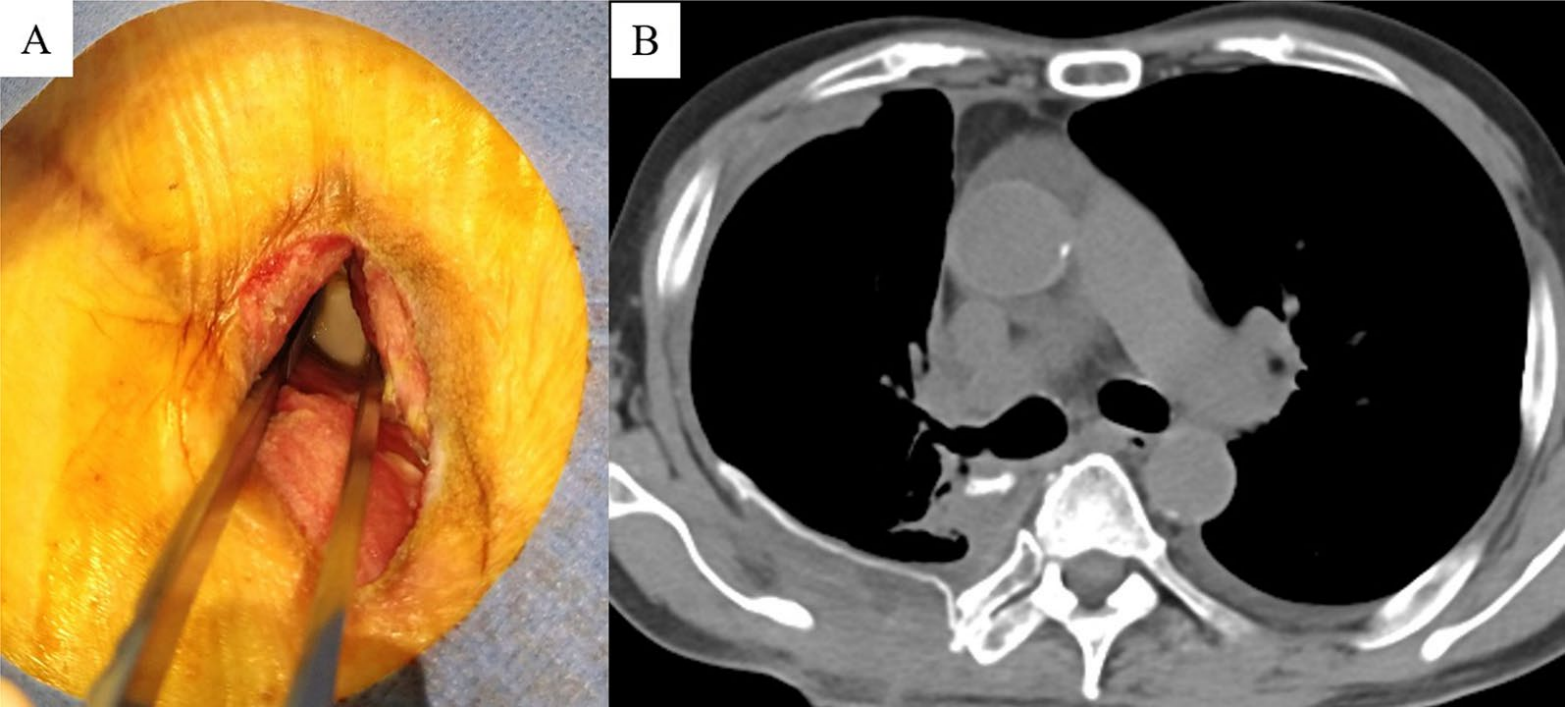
A Formation of wound granulation progressed after VAC therapy. B There was no renewed increase in the space around the PTFE sheet on chest CT
